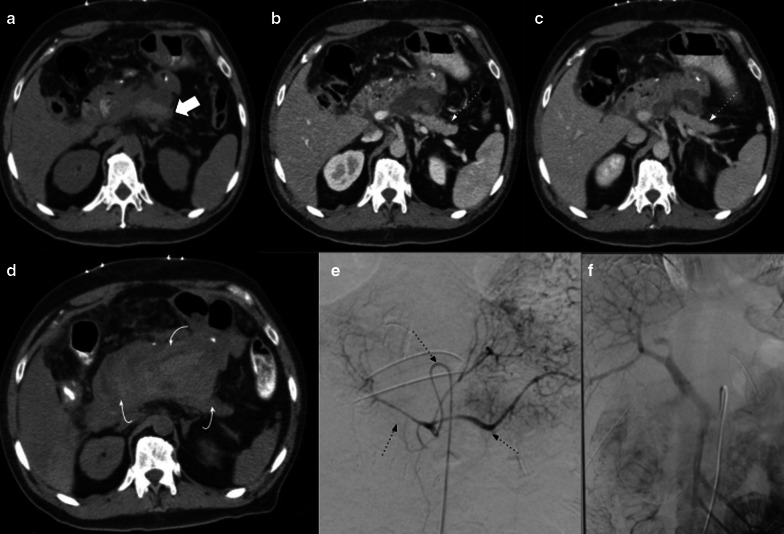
Postoperative hemorrhage. 67-year-old patient during early complicated postoperative. a–c Axial non-contrast, arterial and portal venous phase, respectively. Shows blood collection in the surgical bed (thick white arrow in (a), no active bleeding was seen. Remnant pancreas was preserved (dotted arrow in b and c). (d) CT scan performed three days later due to decreasing hematocrit shows known hematic collection increased in size (curved arrows). (e, f) Digital angiography shows no signs of active bleeding, but there is diffuse spasm of the celiac trunk (dotted black arrows in e) Portal-mesenteric veins were preserved. Exploratory laparotomy confirms hemoperitoneum with retroperitoneal branch-dependent bleeding
